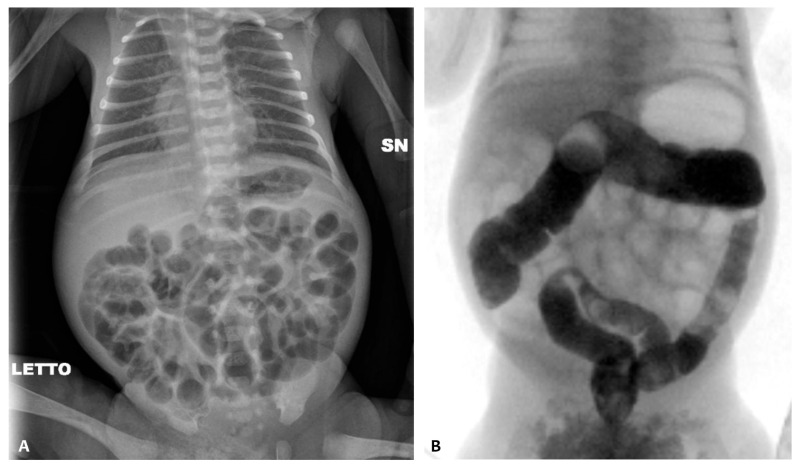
Abdominal X-ray of patient P5 on the 6th day of life (A); barium enema before the NAC treatment (B).
